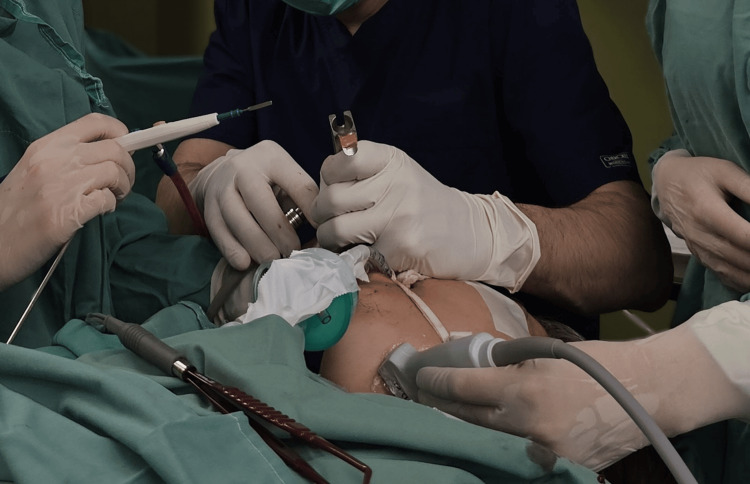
Ultrasound imaging used for localization of the abscess cavity.
